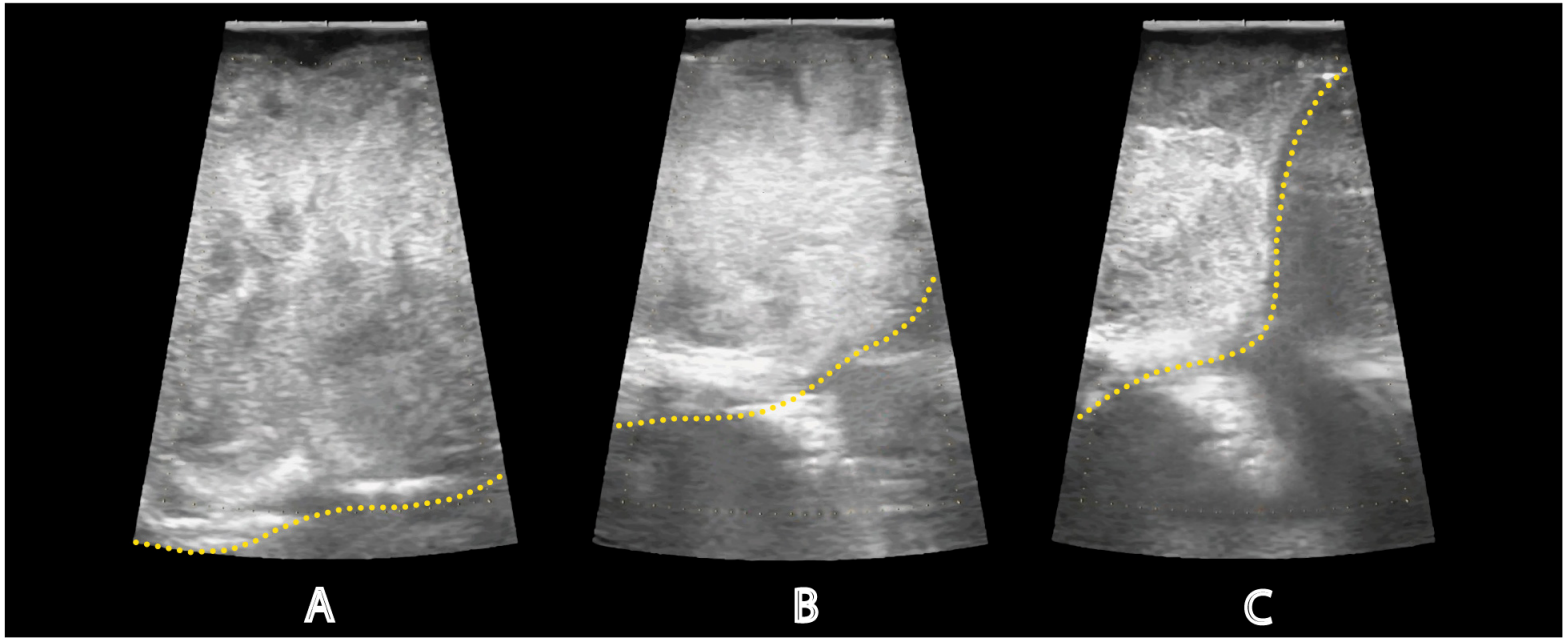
Assessing the extent of tumor resection. Intraoperative ultrasound images show the progressive reduction of remaining tumor tissue throughout resection. The probe is located in the lower clival region and directed toward the patient’s left side. The yellow dotted line denotes the shifting tumor margin. (A) IOUS from the beginning of resection showed a large amount of tumor tissue. IOUS images were taken after twenty (B) and forty-five (C) minutes to assess the extent of resection. IOUS at the end of resection revealed a significant reduction in tumor volume; however, residual tumor was noted laterally and could not be reached endoscopically. The doppler channel was removed in these images for clarity.
